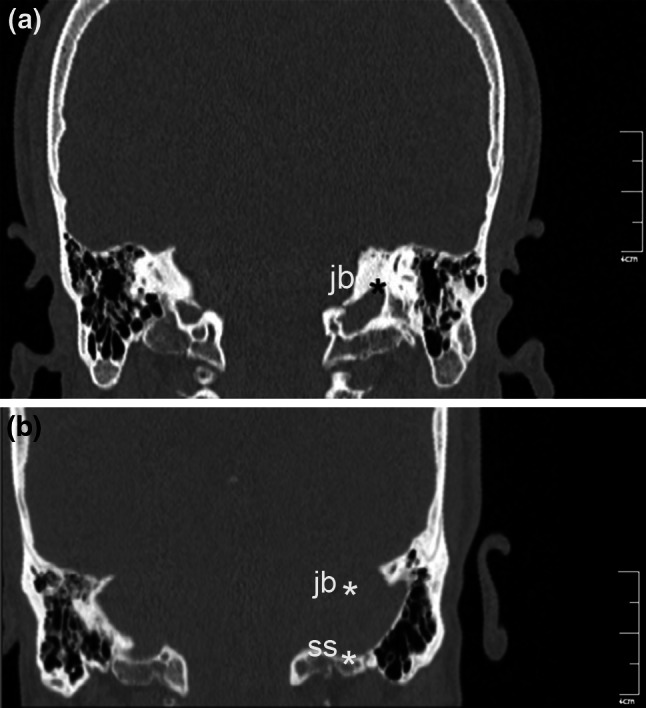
Reformatted coronal computed tomography images. a The *cursor* is on the highest point of the jugular bulb. b The deepest point of the sigmoid sinus (*ss*) is noted, and the height of the jugular bulb (*ss-jb*) is the distance between level of the jugular bulb dome and a *line* passing through confluence of the sigmoid sinus (*ss*) with jugular bulb
